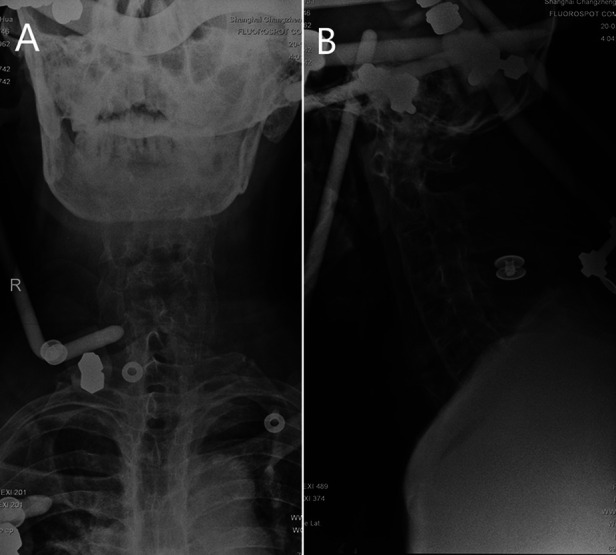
Plain radiographs of the cervical spine revealed closed fracture reduction was performed with the use of a halo-vest (A,B).
